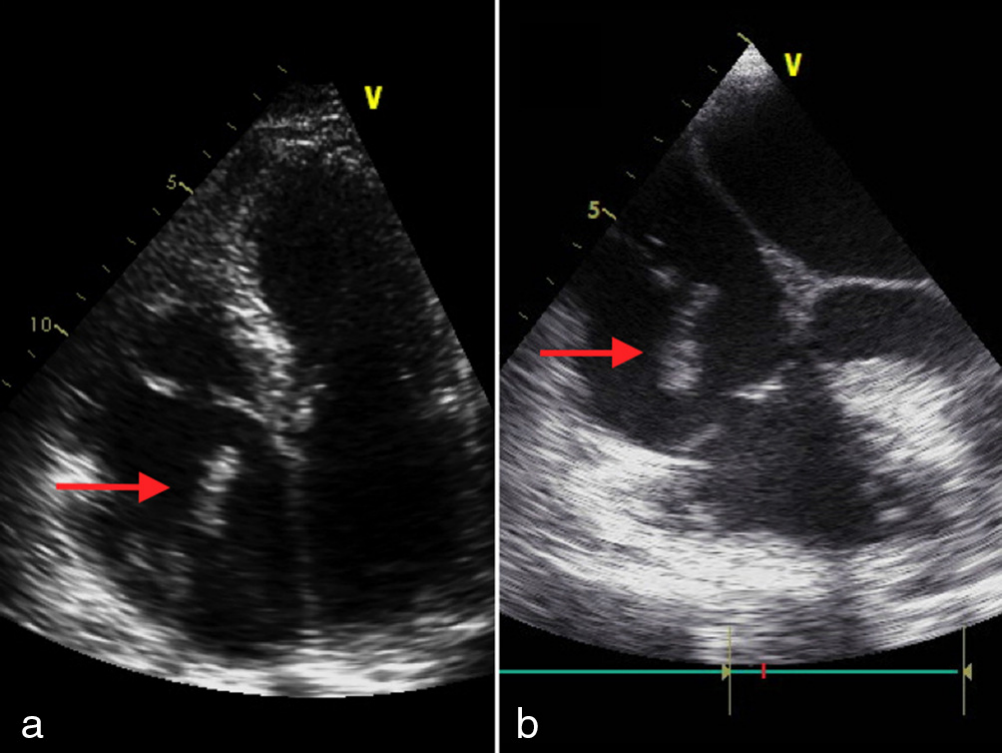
(a) Transthoracic echocardiogram (apical four-chamber view) showing a highly mobile thrombus in the right atrium attached to the pacing lead. (b) Transoesophageal echocardiogram (bicaval view) showing a highly mobile thrombus in the right atrium attached to the pacing lead.
